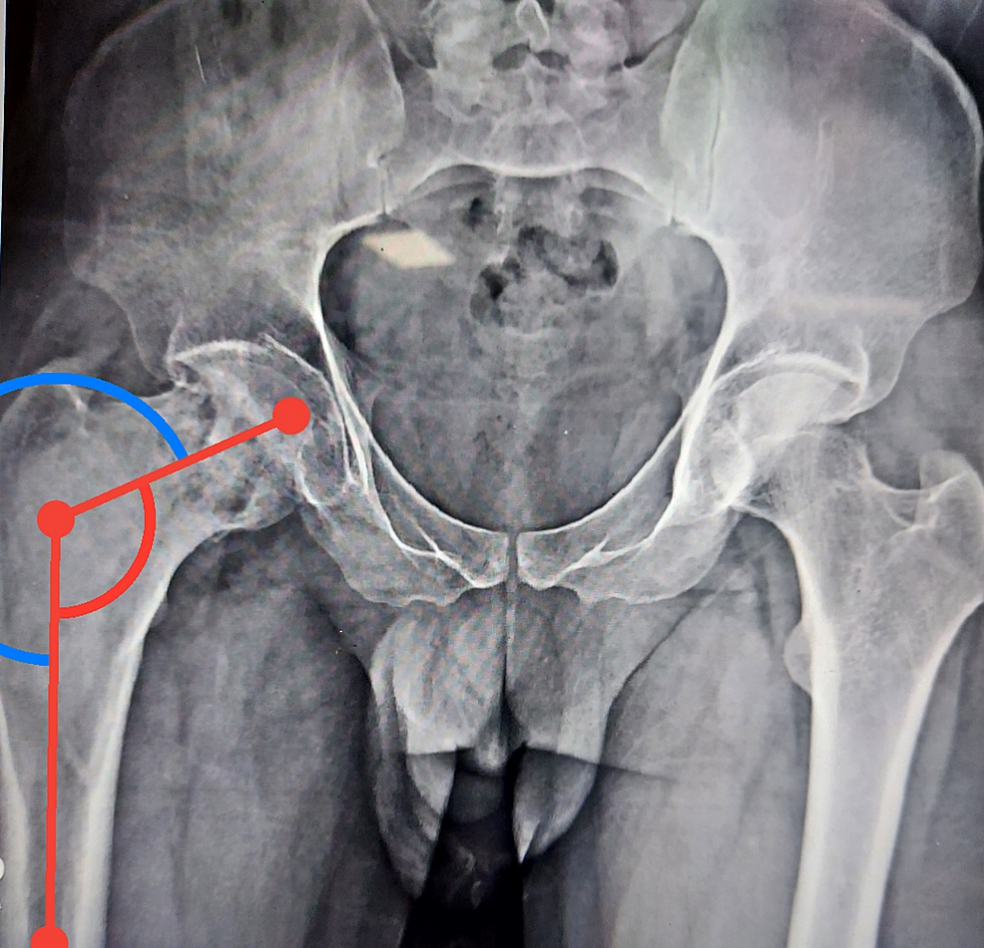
Preoperative x-ray showing Shepherd's crook deformity with neck-shaft angle of 114 degrees
